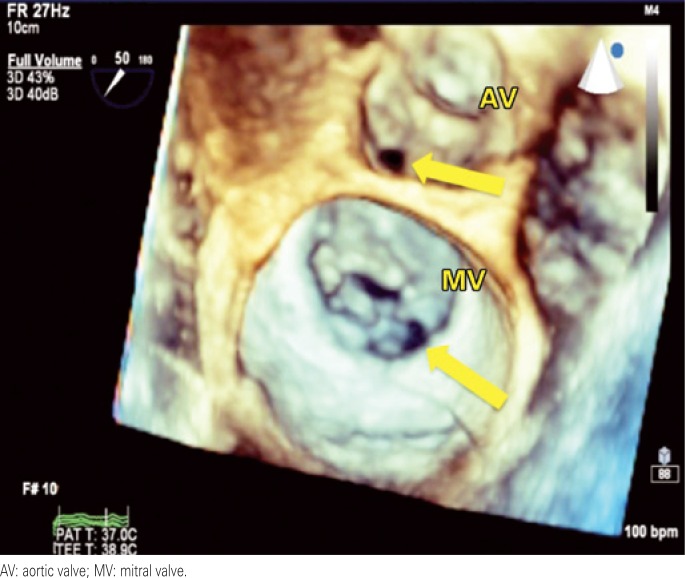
Three-dimensional transthoracic echocardiogram depicting mitral and aortic perforations (arrows)
